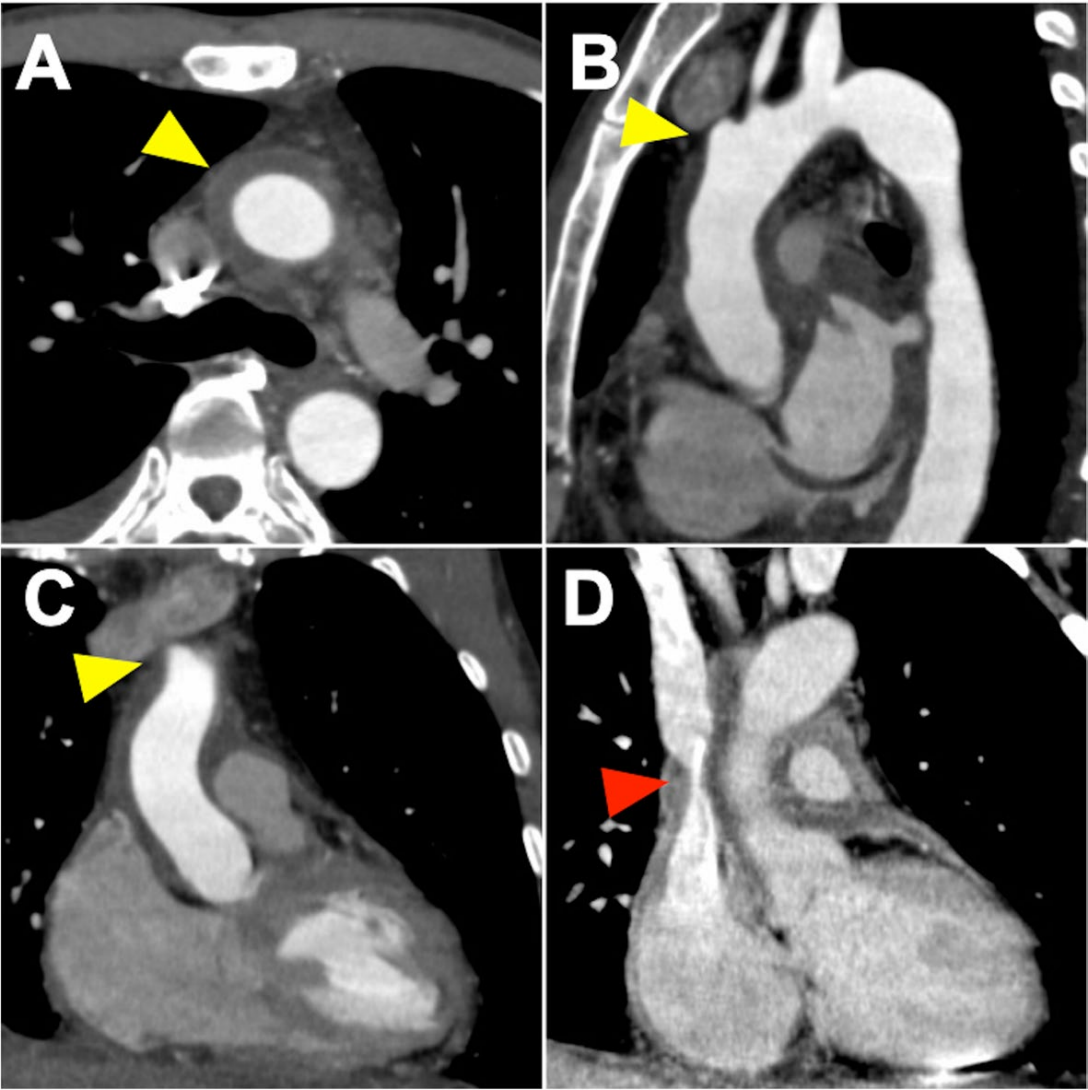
Preoperative enhanced computed tomography. The thrombosed dissection-like finding was spread from the ascending aorta (A yellow arrow) to the proximal aortic arch and the orifice of the right subclavian artery (B, C yellow arrows). The superior vena cava was severely shrunken next to the ascending aorta (D red arrow)
